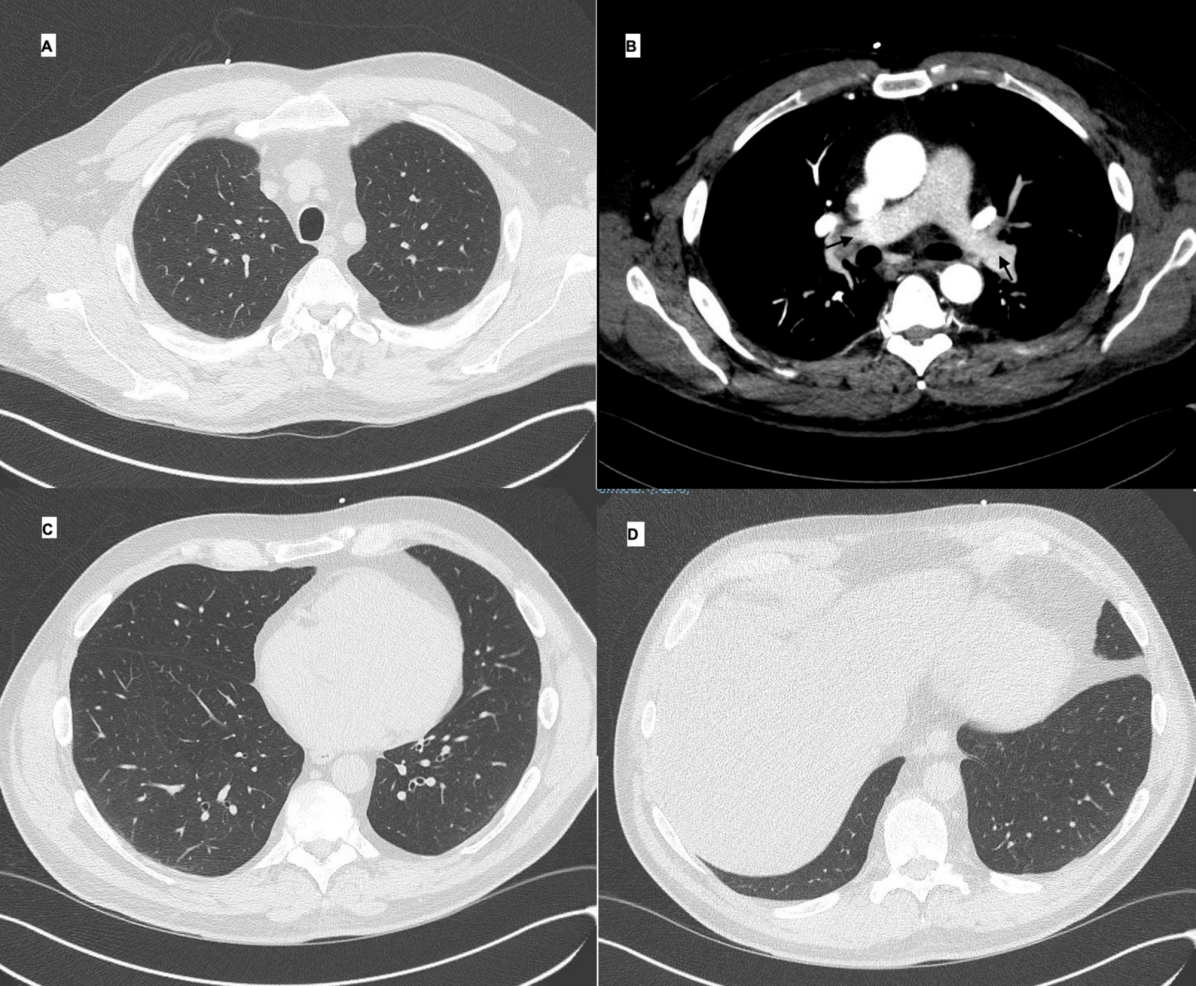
Computed tomography angiography of the chest showing no evidence of pulmonary embolism in major pulmonary arteries (B) and normal lung parenchyma (A, C, and D).
